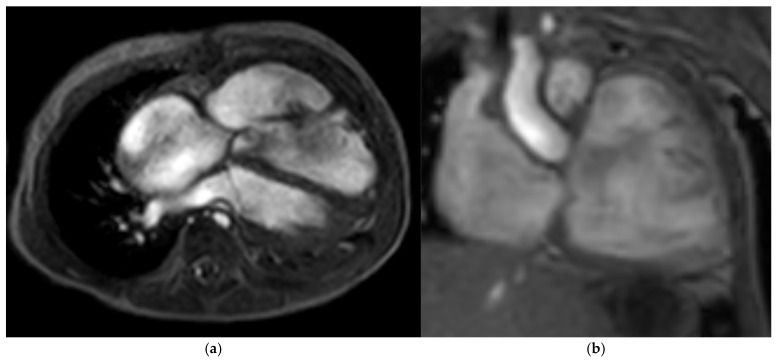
MRI of the right heart and the tricuspid valve; the right atrium and right ventricle present with distinct dilatation; (a) axial plane, (b) coronal plane; 14 months after cone reconstruction, age 2 and a half years.
